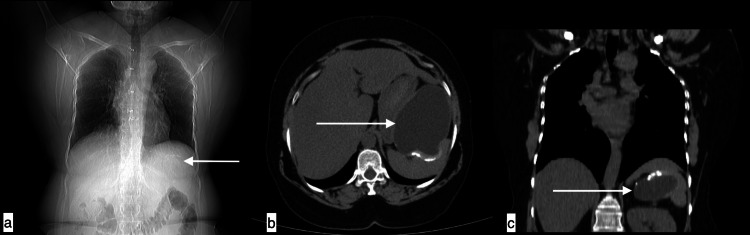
Mesenteric hydatid cyst A 56-year-old male with mesenteric hydatid cyst presented with left upper quadrant pain increasing with exertion for four years. Topogram image (a), axial image (b), and coronal image (c) of non-contrast CT chest revealed a well-defined low attenuating fluid density consistent with thick-walled cystic lesion with dense peripheral calcifications noted in the left upper quadrant, which is seen arising from mesentery, causing indentation over stomach medially and spleen posteriorly.
